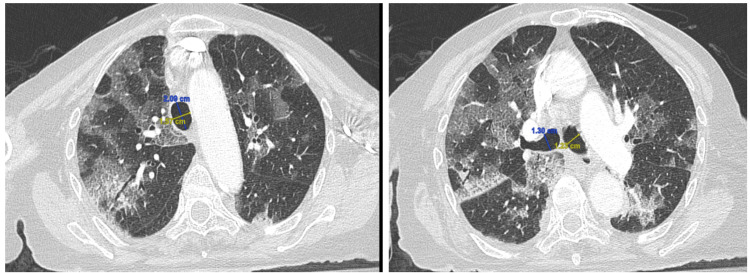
Computed tomography (CT) of the chest highlighting the sagittal (blue) and coronal (yellow) diameters of the trachea (left) and at the level of tracheal bifurcation (right) highlighting the diameter of the right and left bronchi
